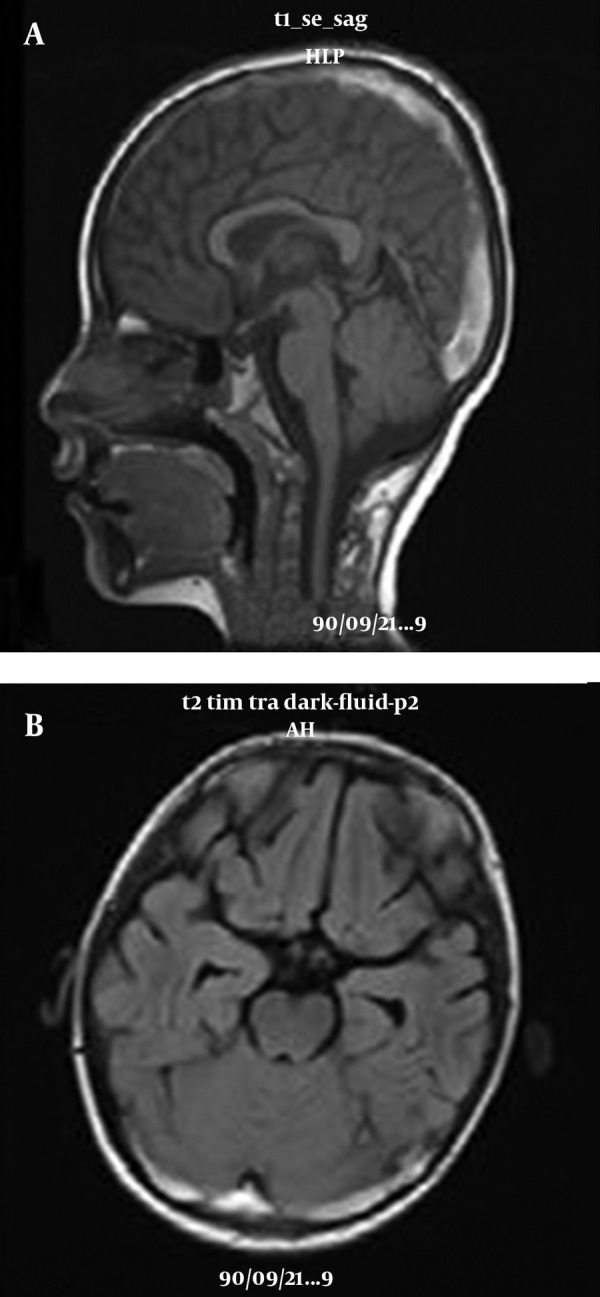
(A), T1-weighted image of brain MRI showed hyper signal intensities in superior sagittal sinus which revealed thrombosis. (B), FLAIR axial MRI showed thrombosis in transverse sinovenouses.
